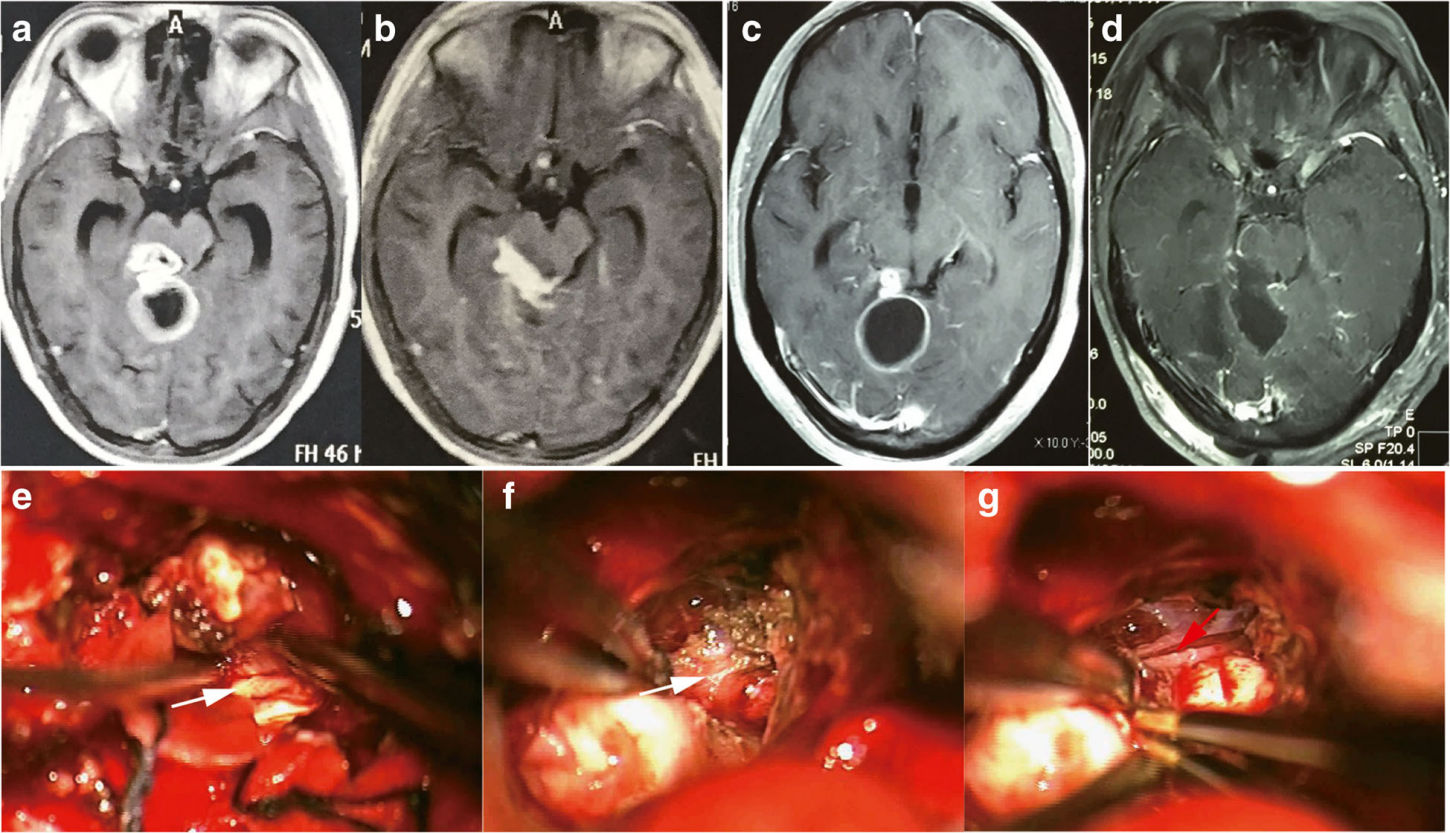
The MRI and intraoperative situation of a tuberculoma associated with ambient cistern. a Preoperative MRI of the lesion. b Drainage surgery was performed for the first time operation at local hospital with residue mainly at the ambient cistern. c The tuberculoma was relapsed with effective ATT and corticosteroids. d A total resection of the lesion was done. e Intraoperative image showed the clear boundary of the part of tuberculoma situating at cerebellum parenchyma (white arrow). f The intracisternal part of the same tuberculoma demonstrated tightly adhesion to the thickening arachnoid which was relatively difficult for separation (white arrow). g Exposure of a vessel coursed in the subarachnoid space (red arrow)
